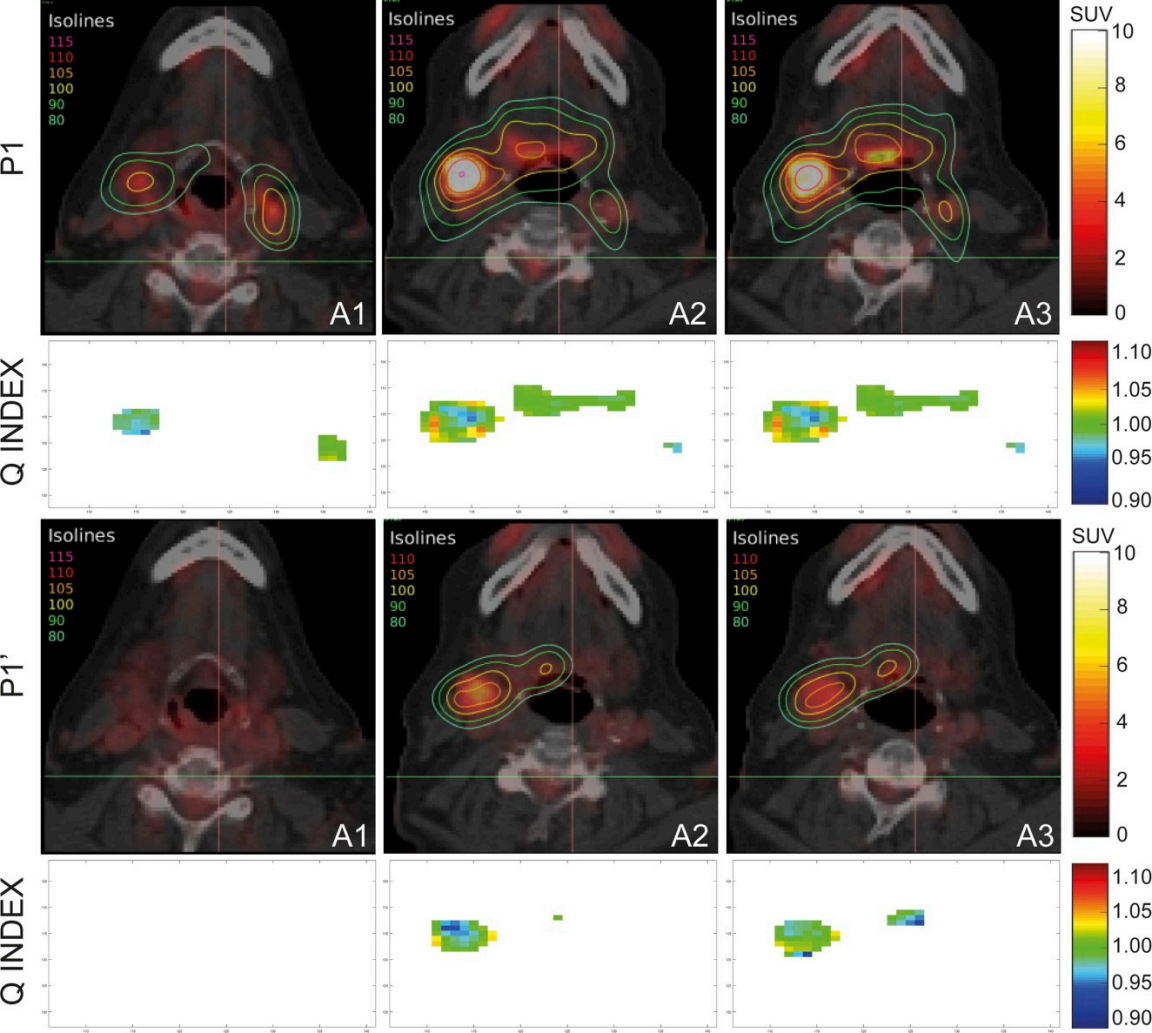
Representative isodose lines as a percentage of the minimum prescription value (70 Gy) corresponding to the plan solutions P1 and P1’ with our DPBN for prescription from initial and follow-up PET/CT image data, respectively. Q index representation is also included.
